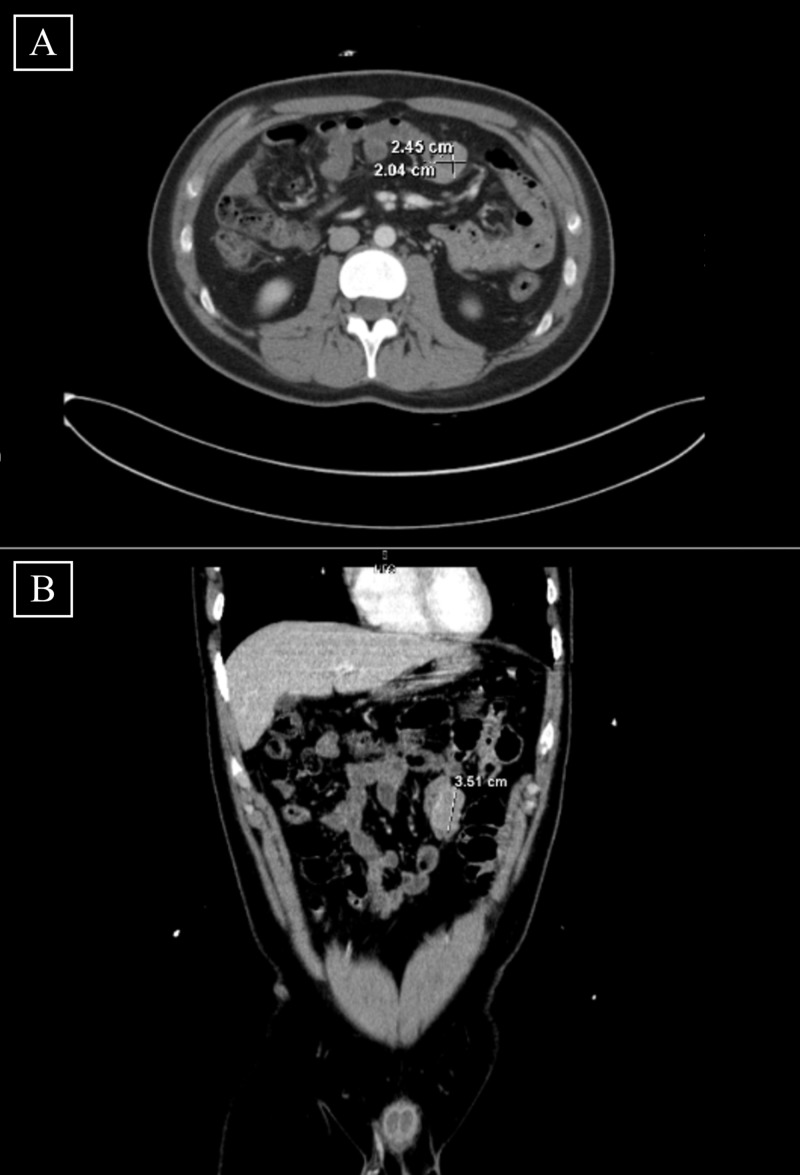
[A] Transverse view of CT scan revealing a 2.45 cm x 2.04 cm well-circumscribed ovoid mass in the jejunum. [B] Coronal view of CT scan revealing a 3.51 cm well-circumscribed ovoid mass in the jejunum. CT: Computed tomography
